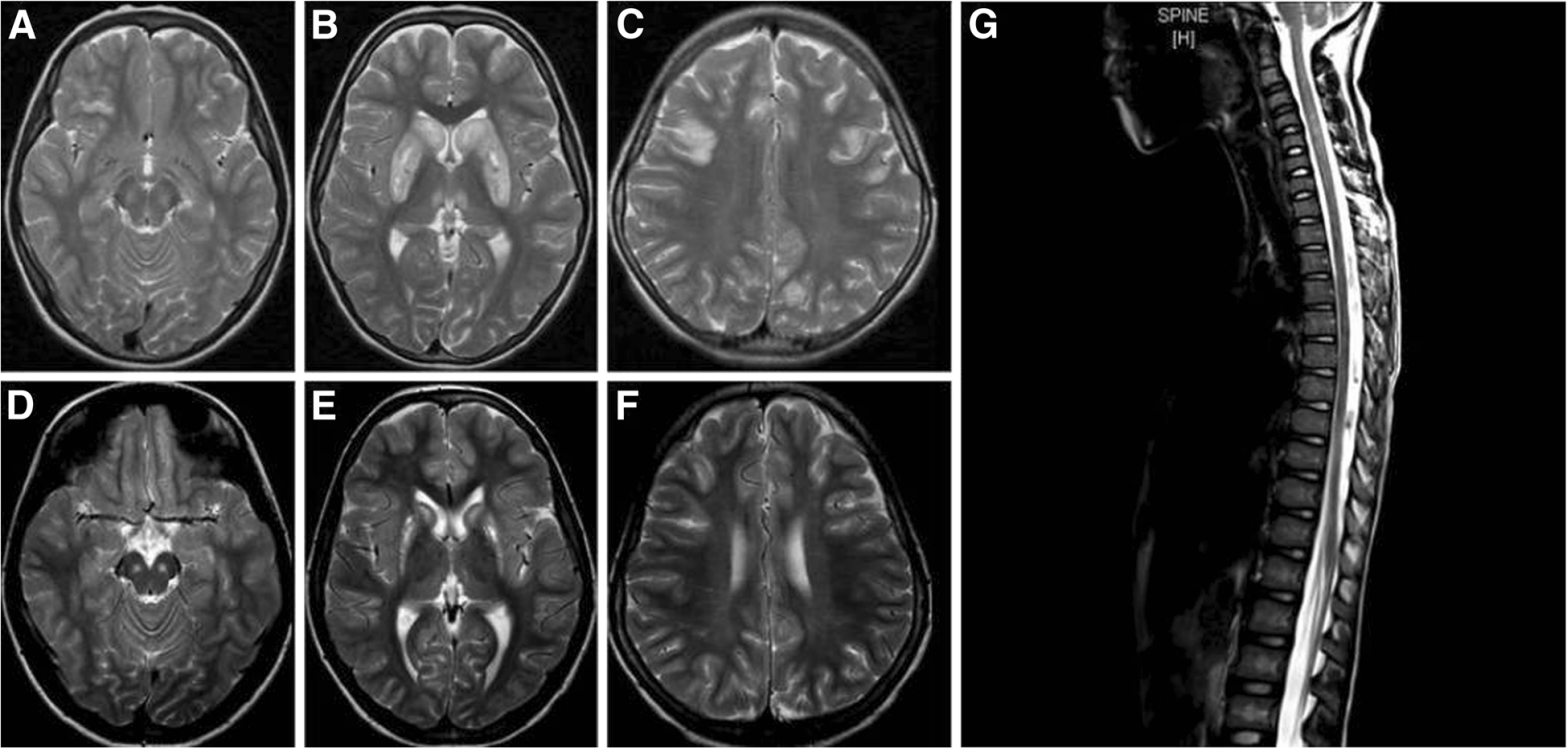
MRI brain axial T2 weighted spin echo images – (images A to F) and MRI spine sagittal T2 weighted image – (image G). Upper column: (A, B, C) was done for the patient during the acute crisis. A: This image shows high T2 signal and swelling of substantia nigra (at midbrain), and signal abnormality with swelling of right inferior frontal gyrus in the cortical and subcortical region. B: It shows high T2 signal and swelling of lentiform, caudate head with small necrotic changes and involvement of medial thalami. C: It shows focal high T2 cortical signal and subcortical white matter signal at both frontal and parietal lobes. Lower column: (D, E, F) was done as a follow up exam for the same patient during chronic stage of the disease. D: This image was done at the level of the midbrain and shows focal high T2 fluid signal in the substantia nigra which evolved from swelling seen during the acute crisis (image A). E: It shows high T2 (fluid signal) and atrophy in the basal ganglia (lentiform and caudate nuclei) indicates evolved swelling into necrosis. F: It shows interval resolution of the cortical and subcortical signal seen during the acute crisis at the frontal lobes. MRI Image of the spine (G) shows patchy high T2 intramedullary signal most conspicuous in the cervical spinal cord with swelling extends from C3 to C7 vertebral level.
